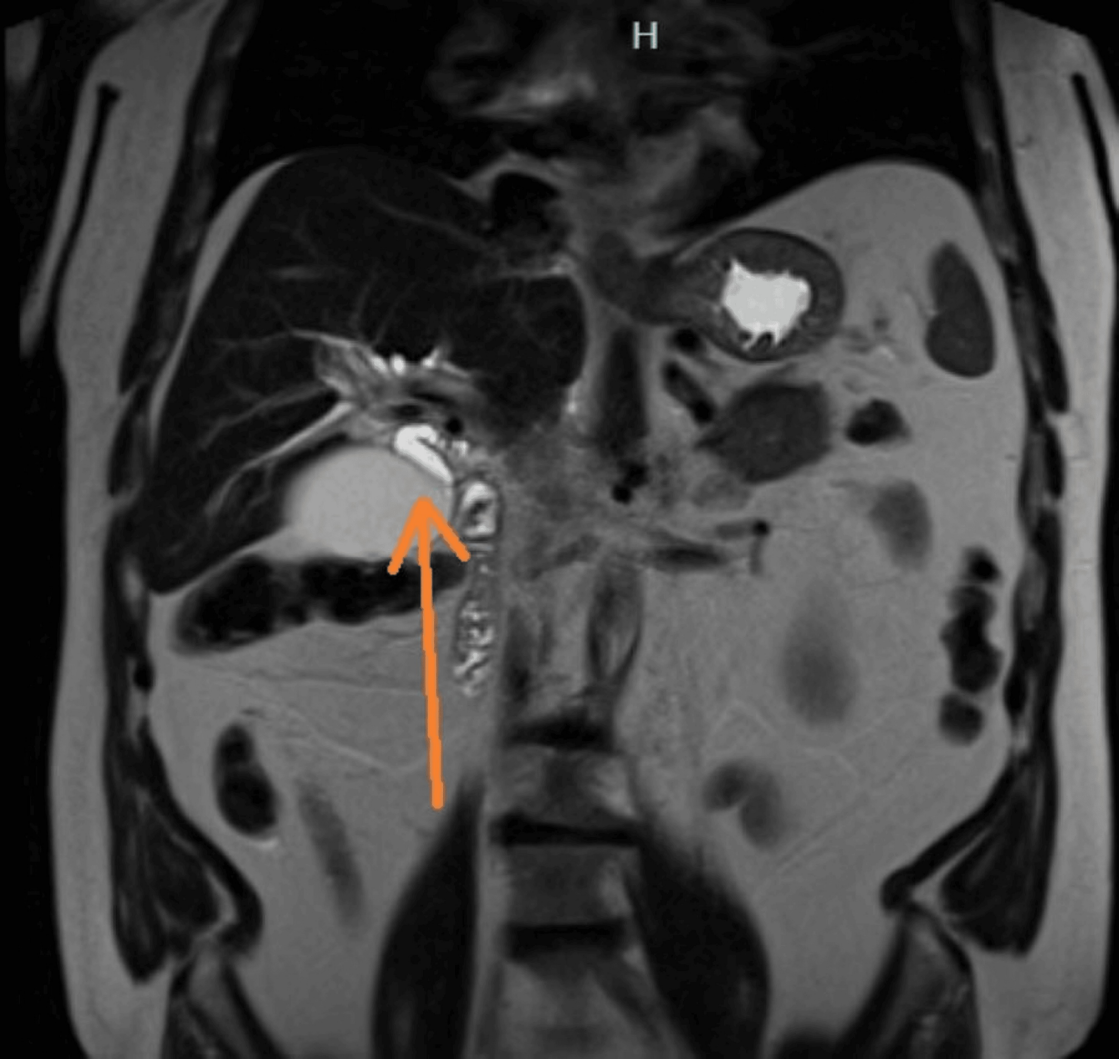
Coronal MRI of the abdomen with MRCP demonstrating a distended gallbladder and a moderately dilated proximal common bile duct (orange arrow).
